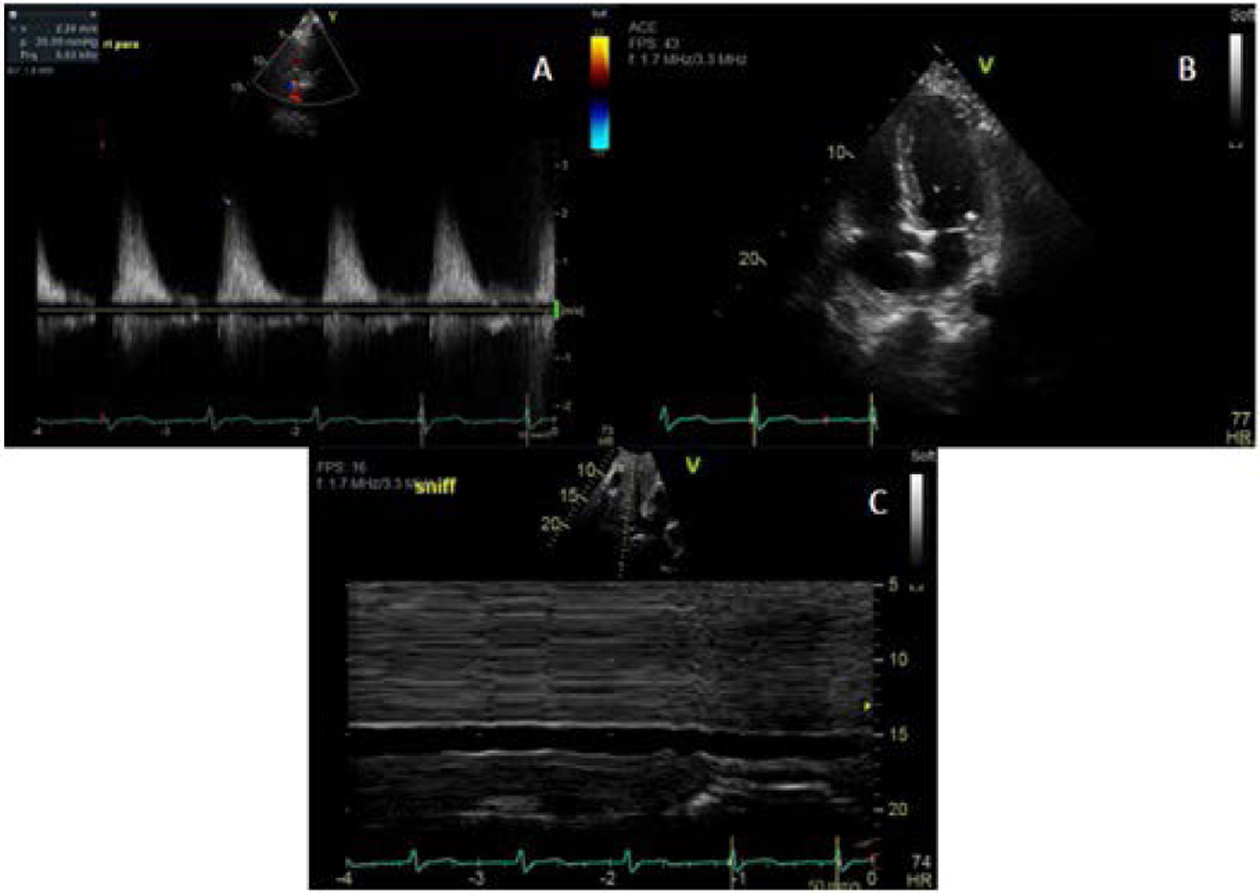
Images A-C Showing Echocardiographic Evidence of Pulmonary Hypertension
